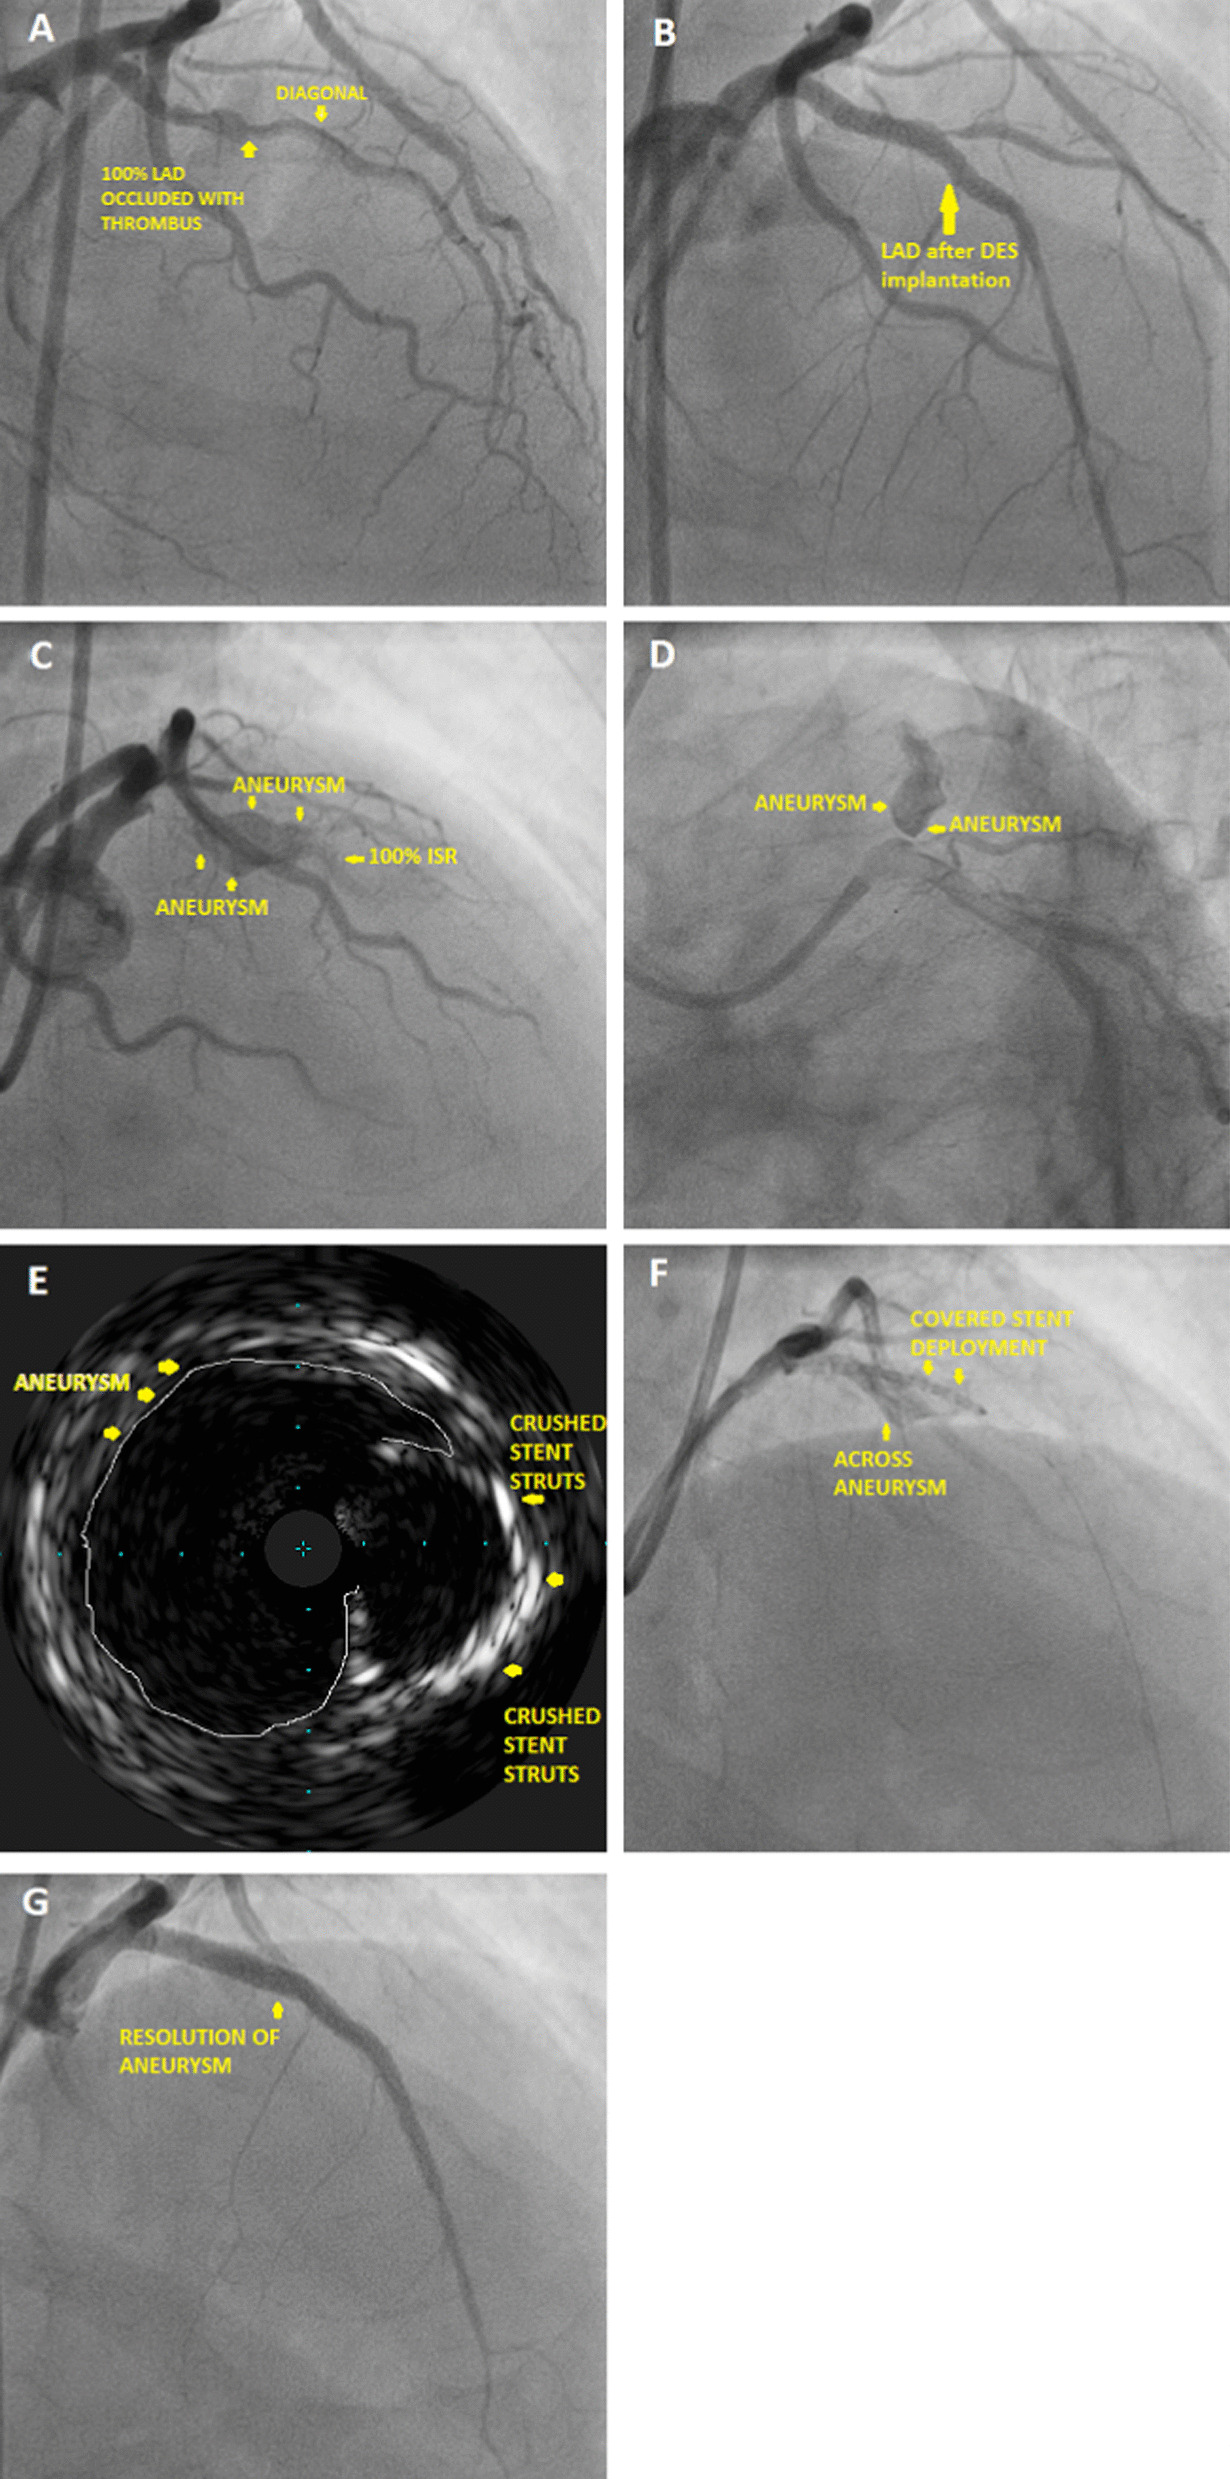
A Coronary angiogram showing mid LAD 100% occluded with thrombus, B post stenting of LAD with DES, C angiogram showing aneurysm in LAD with 100% ISR distally, D angiogram showing coronary aneurysm in LAD, E intravascular ultrasound showing crushed stent struts with adjoining aneurysm, F covered stent deployment across the aneurysm, G after covered stent deployment resolution of aneurysm
